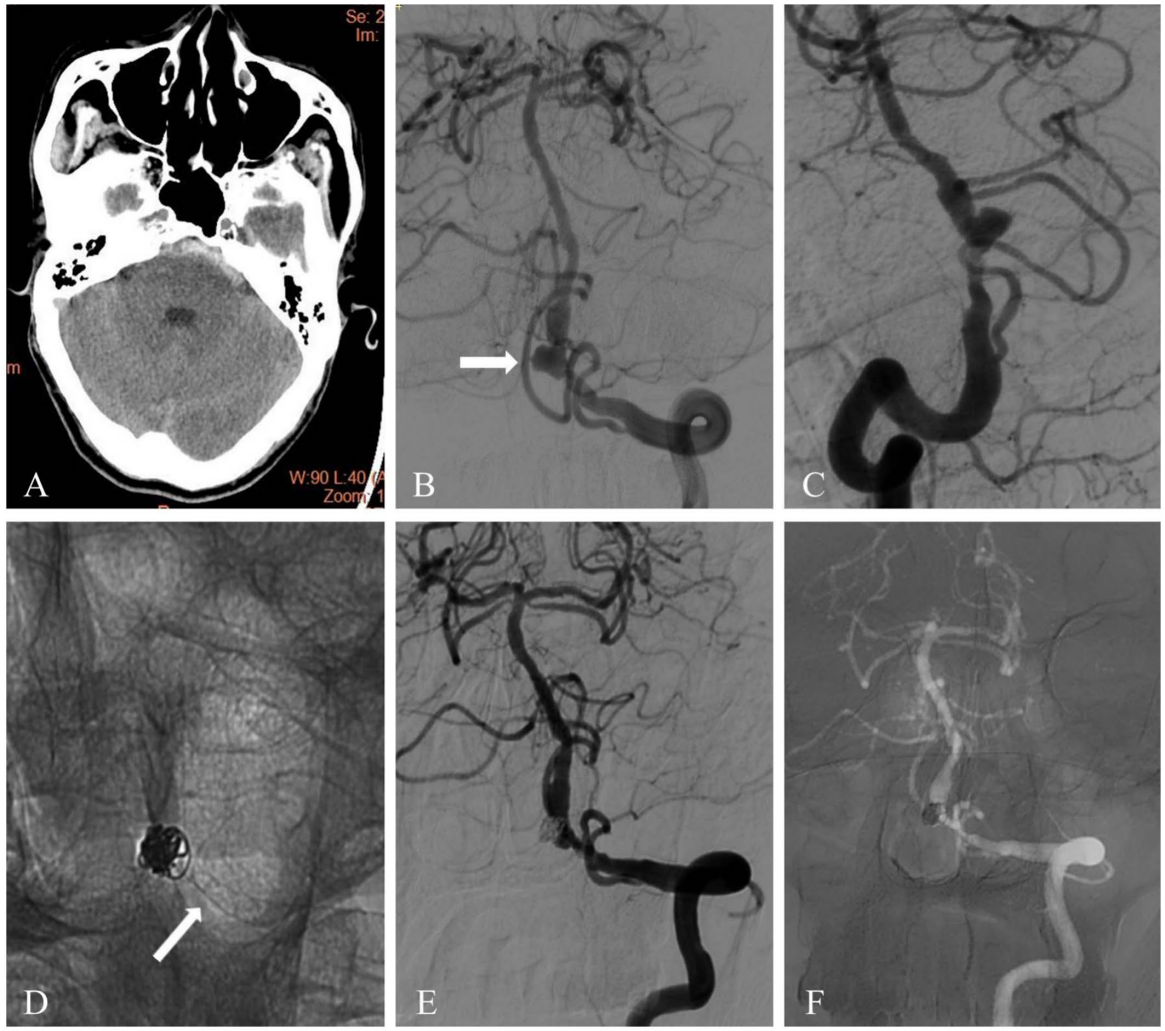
A 61-year-old patient presented with subarachnoid hemorrhage and a vertebrobasilar junction dissecting aneurysm. (A) Head computed tomography showed acute subarachnoid hemorrhage. (B,C) Diagnostic cerebral angiography revealed a dissecting aneurysm located in the vertebrobasilar junction (white arrow). (D) The dissecting aneurysm was treated with coiling and a PED. Unsubstracted angiogram showed the flow diverter (white arrow) and coil mass. (E) Immediate post-treatment angiography showed complete occlusion of aneurysm and patency of parent vessel. (F) Follow-up angiography at 8 months showed complete occlusion of aneurysm and parent vessel patency.
